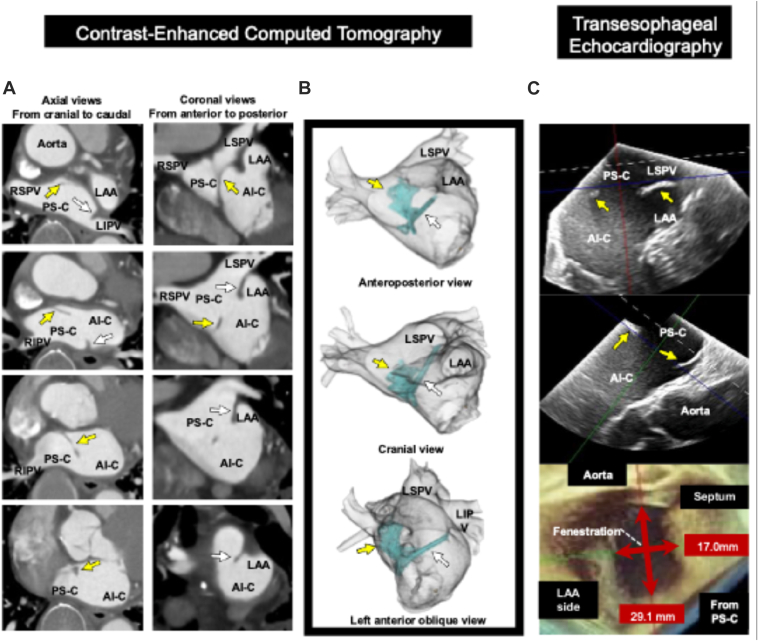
Preablation imaging of the LA membrane and fenestration. A: Contrast-enhanced computed tomography showing the LA membrane. B: 3-dimensional reconstruction of contrast-enhanced computed tomography demonstrating the LA membrane. C: Transesophageal echocardiography identifying the LA membrane and fenestration (17.0 × 29.1 mm). *Yellow and white arrows*: LA membrane. AI-C = anteroinferior compartment; LA = left atrium; LAA = left atrial appendage; LIPV = left inferior pulmonary vein; LSPV = left superior pulmonary vein; PS-C = posterosuperior compartment; RIPV = right inferior pulmonary vein; RSPV = right superior pulmonary vein.
